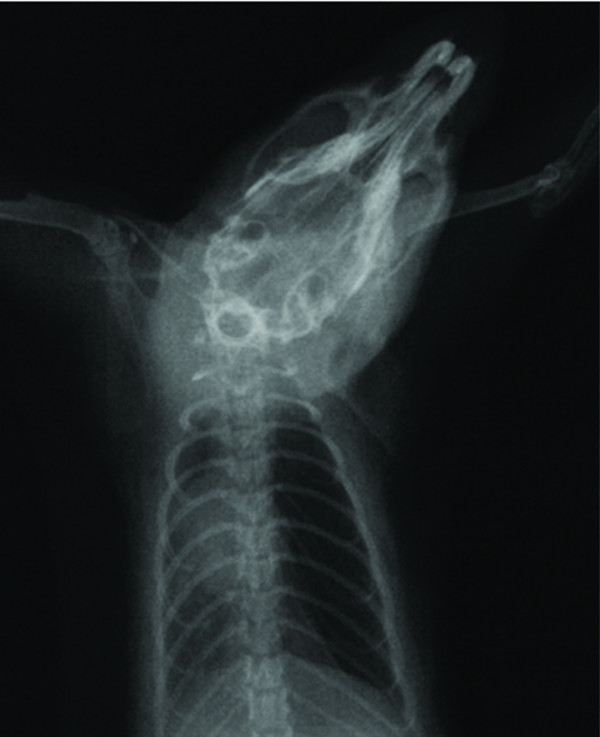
The confirmation of right re-expansion by chest X-ray. Re-expansion pulmonary edema is also seen.
